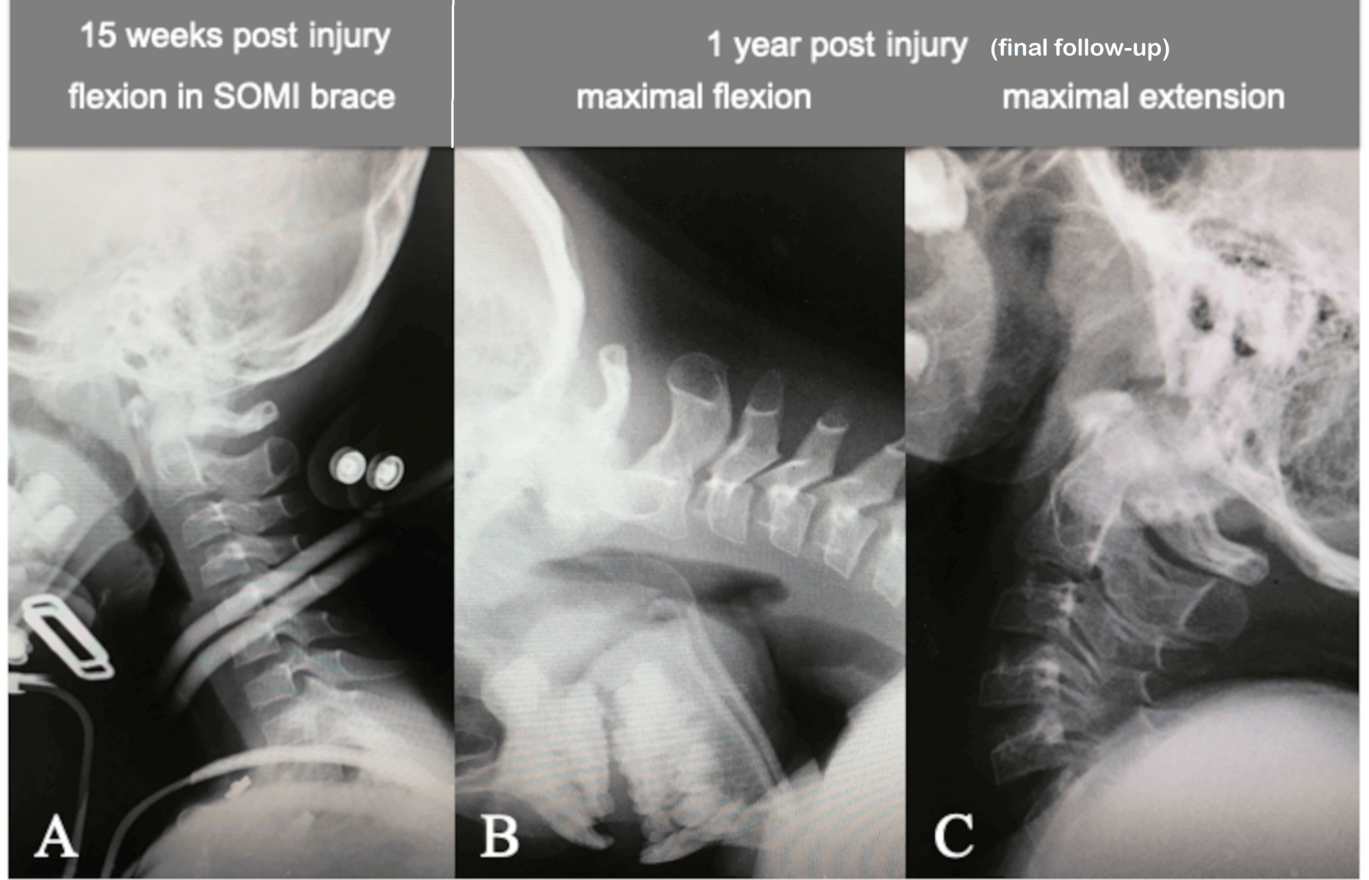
Cervical spine X-rays at 15 weeks post injury and at the one-year final follow-up Dynamic flexion-extension cervical spine radiographs showing (A) a normal atlantodental interval (3 mm) and no evidence of instability at 15 weeks post injury and (B, C) that the cervical ROM remained normal with no abnormal motion or instability at the final one-year follow-up. ROM: range of movement; SOMI: sternal occipital mandibular immobilizer
